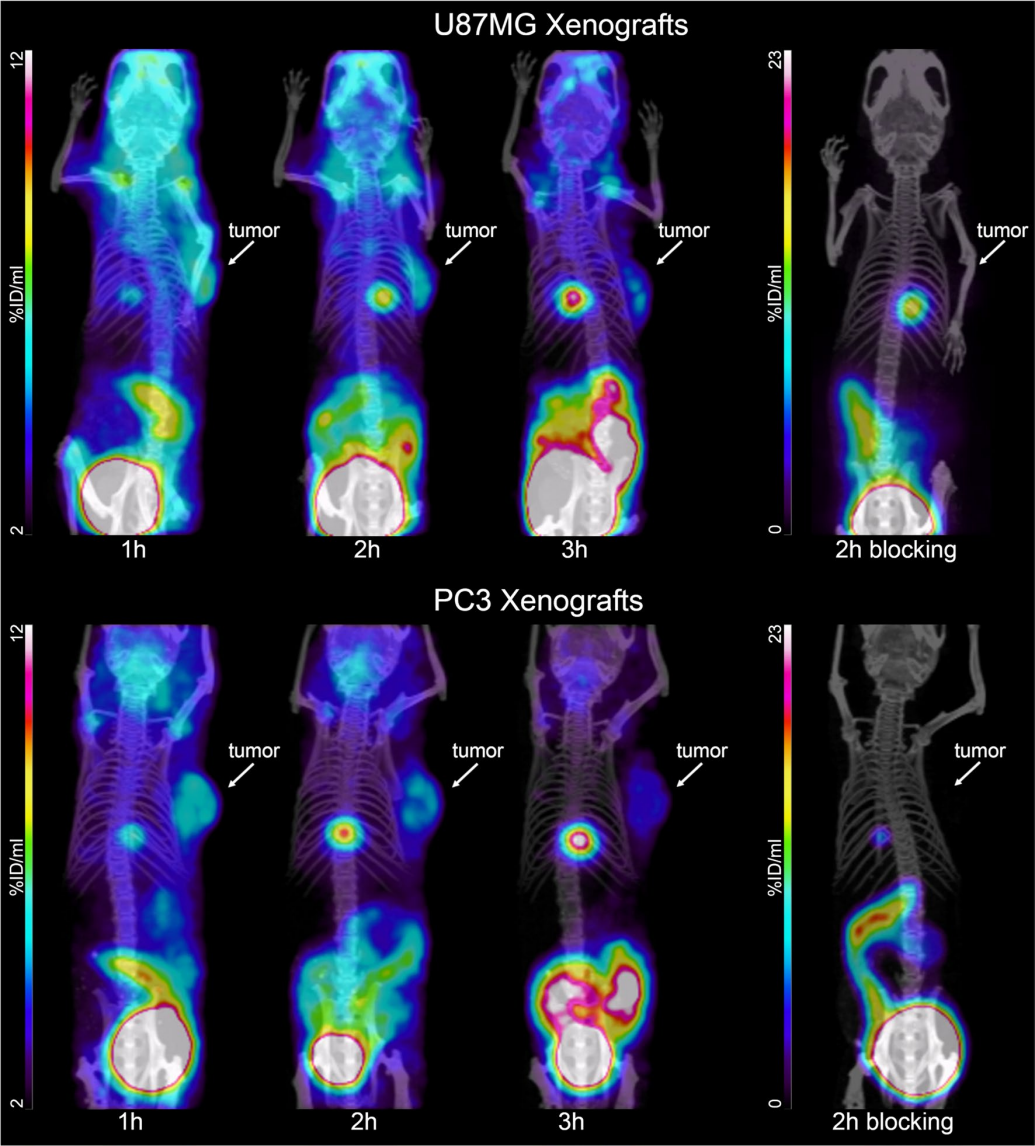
PET/CT images of U87MG and PC3 xenografts upon injection of [^68^ Ga]Ga-DATA^5m^.SA.FAPi at 1, 2 and 3 h along with blocking studies at 2 h after injection
